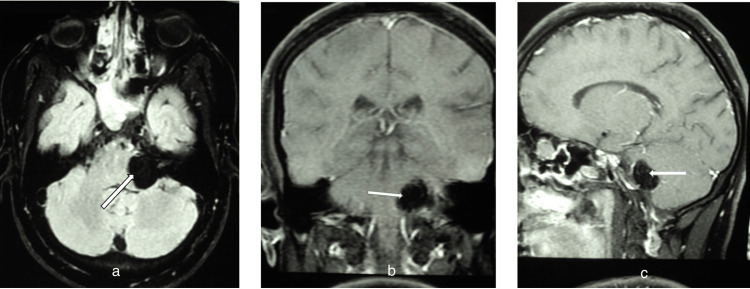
Post-contrast fat saturation sequence MRI a) Axial view showing fat suppression images presenting characteristics of fatty nature of the left cerebellopontine angle (CPA) lesion, which are confirmed in b) coronal and c) sagittal sections.
